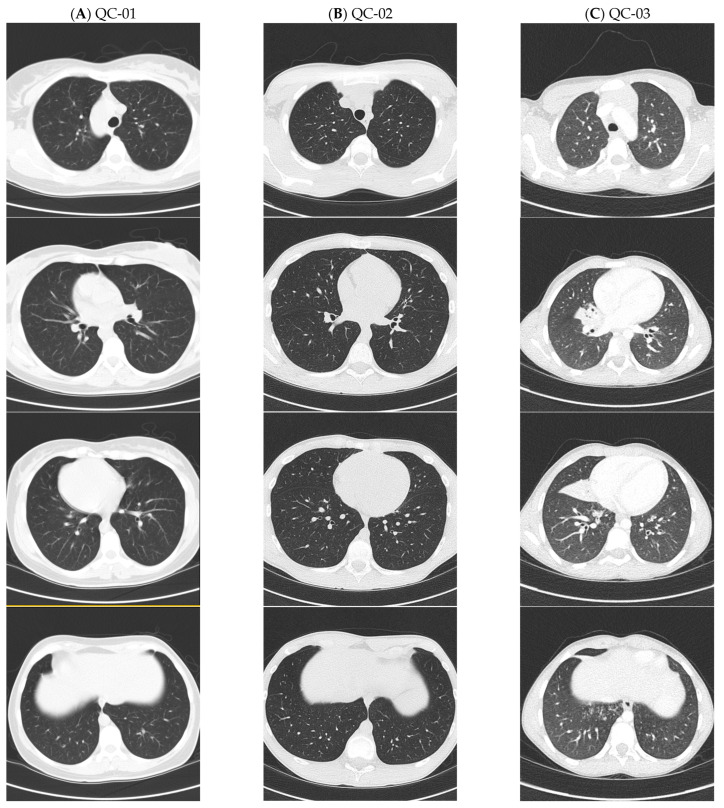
Lung CT scan images from patients with *ODAD4*-related PCD. Caption: (A) normal lung CT images with absence of bronchiectasis in QC-01 at 38 years old, (B) normal lung CT images with absence of bronchiectasis in QC-02 at 14 years old, and (C) right middle lobe atelectasis with bronchiectasis seen on lung CT images of QC-03 at 8 years old.
